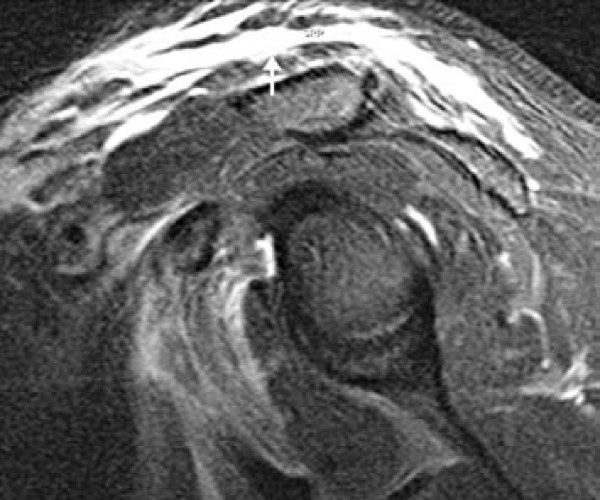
Sagittal oblique fat-suppressed post contrast MRI left shoulder (TR/TE 5670/75). There is extensive subcutaneous oedema in the soft tissues superficial to the deltoid and rotator cuff muscles (arrow), consistent with left shoulder fascitis. The underlying supraspinatous tendon and muscles are normal.
